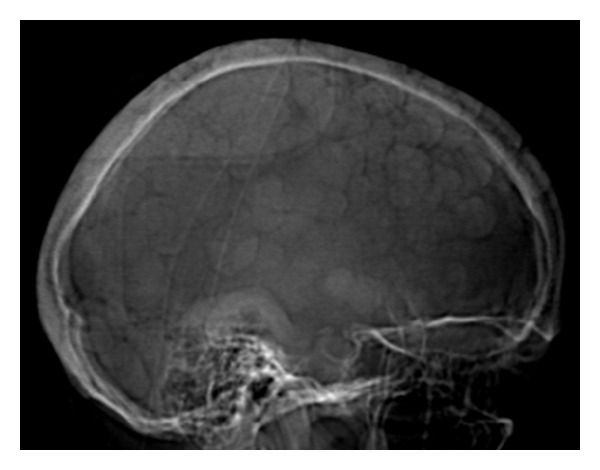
Skull radiograph, lateral view: multiple nodular densities overlie the calvarium.
